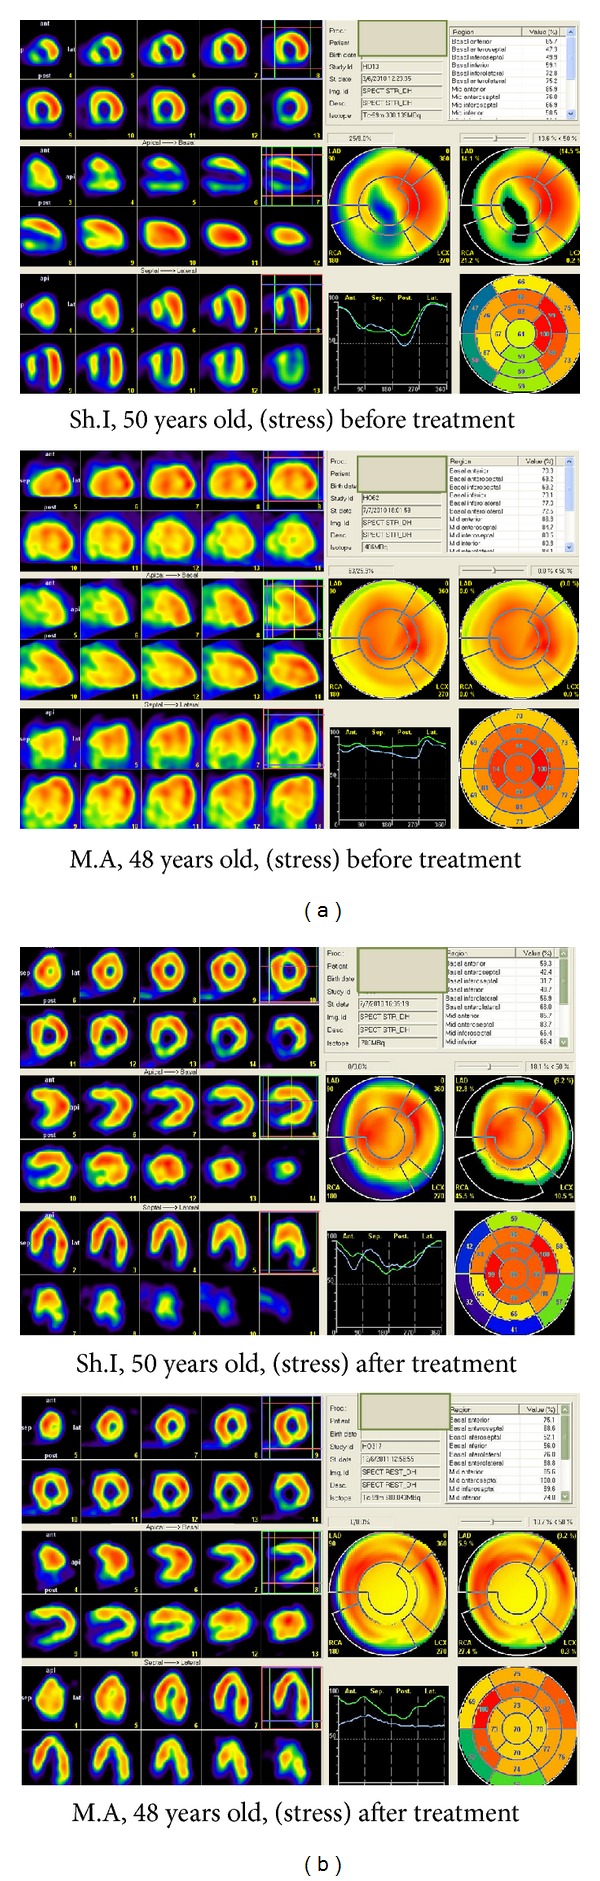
The areas that benefit from myocardial revascularization after single cell therapy in two patients, carried out by myocardial scintigraphy (post stress condition) before treatments and after 9 months (black, no perfusion; blue-green-yellow-red, increasing perfusion).
